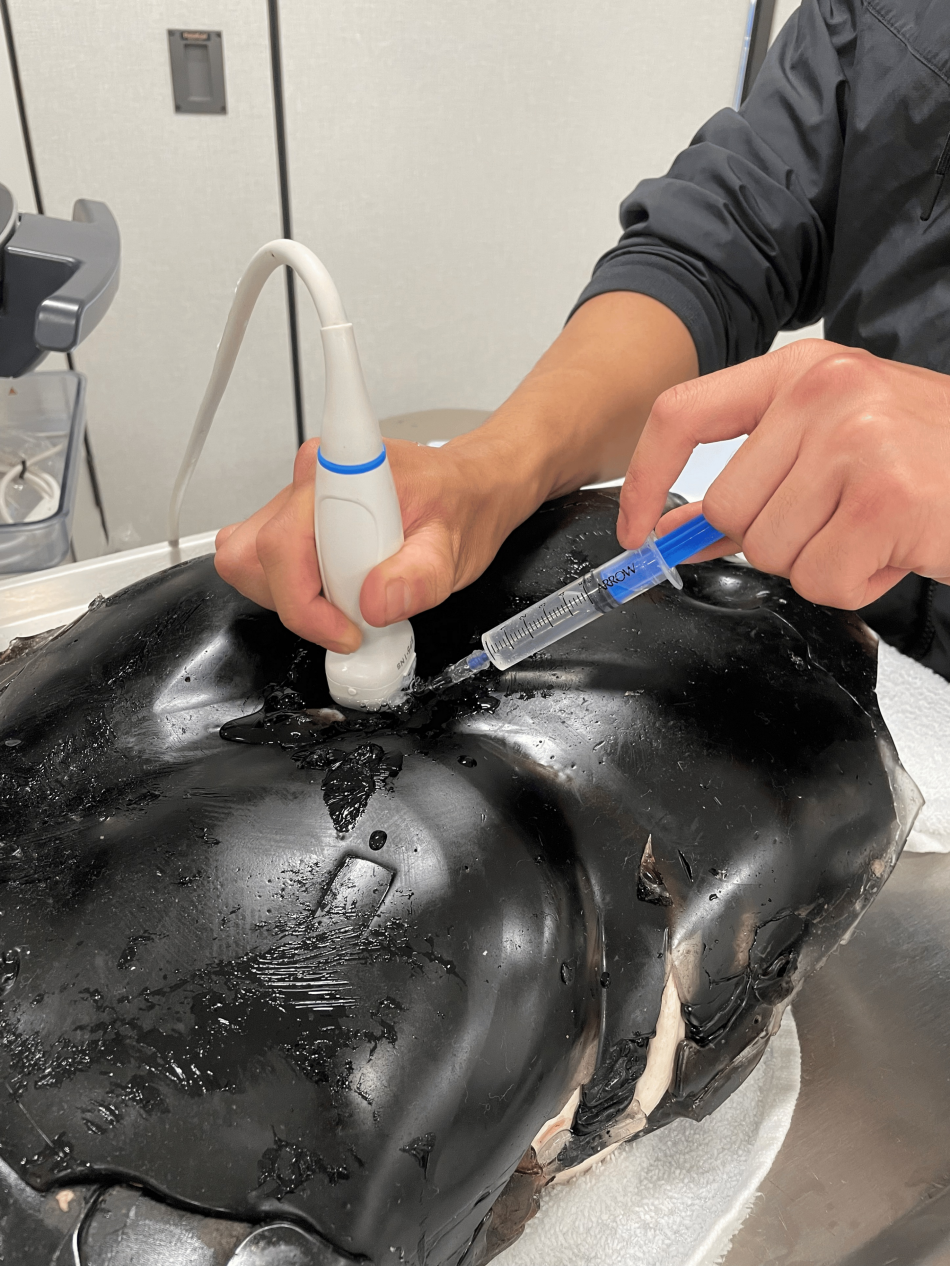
Model In Use With Ultrasound Guidance
